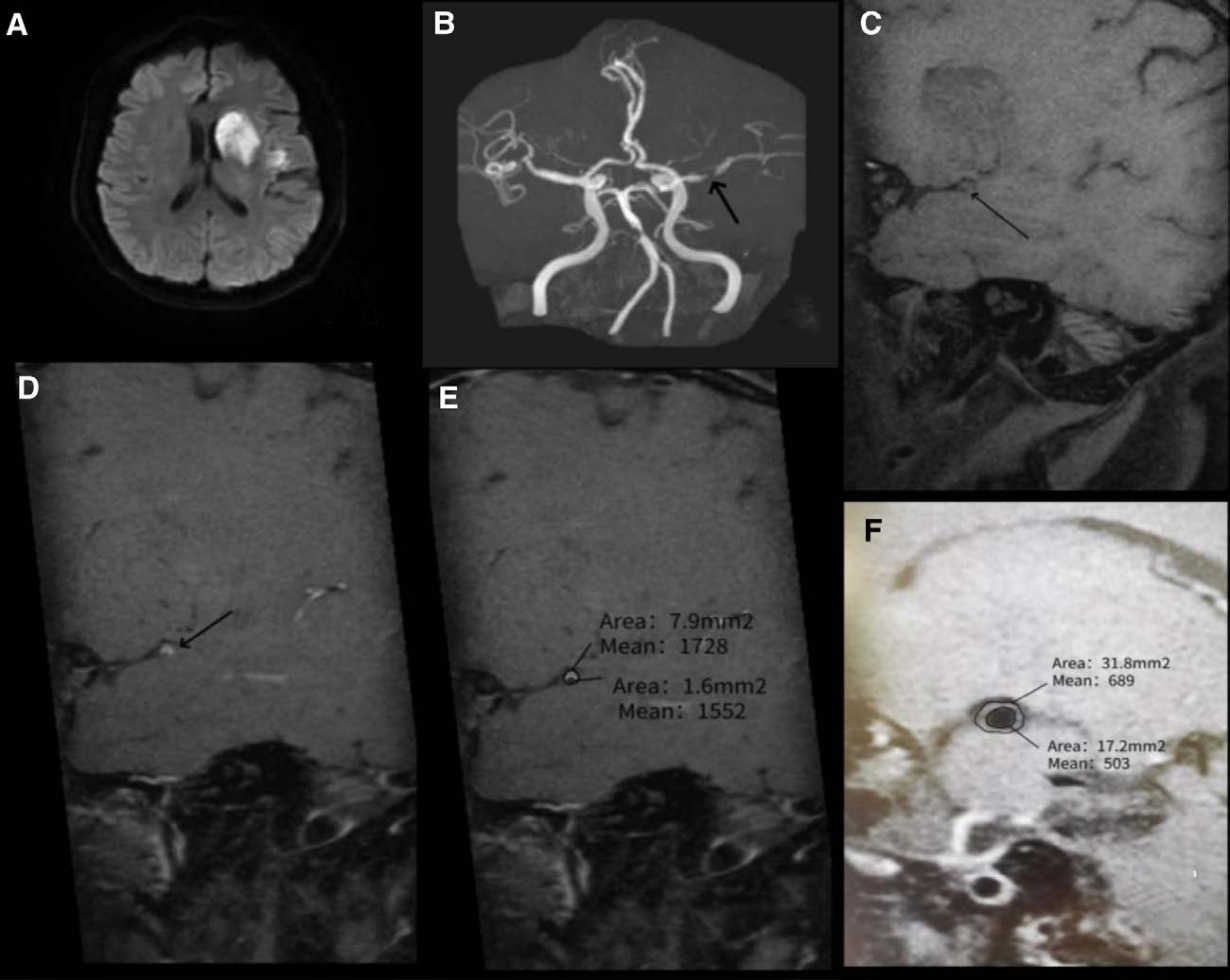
HR-MRI of a symptomatic MCA stenosis in a 54-yr-old male who presented with dysphonia for 1 d and right limb weakness for 10 h. (A) DWI indicates an AIS in the distribution of the left MCA. (B) TOF-MRA demonstrates severe stenosis of the M1 segment of the left MCA (white arrow). (C) The plaque is visible on T1-weighted HR-MRI (black arrow). (D) T1-weighted post-contrast HR-MRI shows eccentric wall thickening with enhancement at the plaque site (black arrow). (E) At the most narrowed site, post-contrast T1-weighted HR-MRI shows a vessel area of 7.9 mm^2^ and an LA of 1.6 mm^2^. (F) At the reference site, the vessel area is 31.8 mm^2^ and the LA is 17.2 mm^2^. The degree of stenosis = (1–1.6 mm^2^/ 17.2 mm^2^) × 100% = 90.7%. AIS = acute ischemic stroke, DWI = diffusion weighted imaging, HR-MRI = high-resolution magnetic resonance imaging, LA = lumen area, MCA = middle cerebral artery, TOF-MRA = time-of-flight magnetic resonance angiography.
